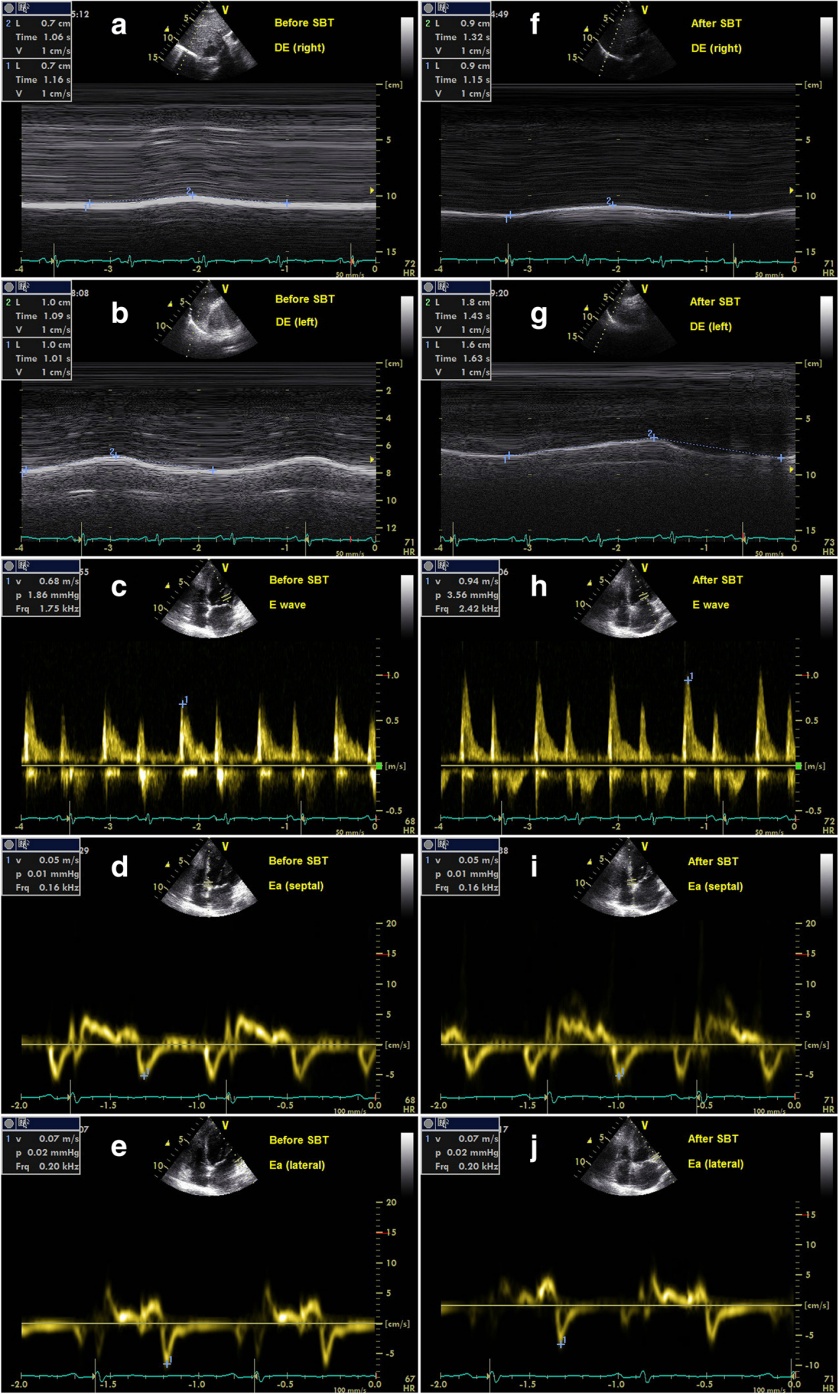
The measurements of E/Ea and DE before and after SBT in a patient of respiratory failure within 48 h. a, b, c, d, e represented the results before SBT, and f, g, h, i, j represented the results after SBT. a DE (right) was 7 mm. b DE (left) was 10 mm. c E was 68 cm/s. d Ea (septal) was 5 cm/s. e Ea (lateral) was 7 cm/s. f DE (right) was 9 mm. g DE (left) was 17 mm. h E was 94 cm/s. i Ea (septal) was 5 cm/s. j Ea (lateral) was 7 cm/s. Before SBT, DE (average) was 8.5 mm, and E/Ea (average) was 11.3. After SBT, DE (average) was 13 mm, and E/Ea (average) was 15.7. After extubation, respiratory failure occurred within 48 h and then NIV was used. The patient was not re-intubated within 1 week and free of NIV in the end. *DE* diaphragmatic excursion, *E/Ea* the ratio of mitral Doppler inflow velocity (E) to annular tissue Doppler wave velocity (Ea), *NIV* noninvasive ventilation, *SBT* spontaneous breathing trial
